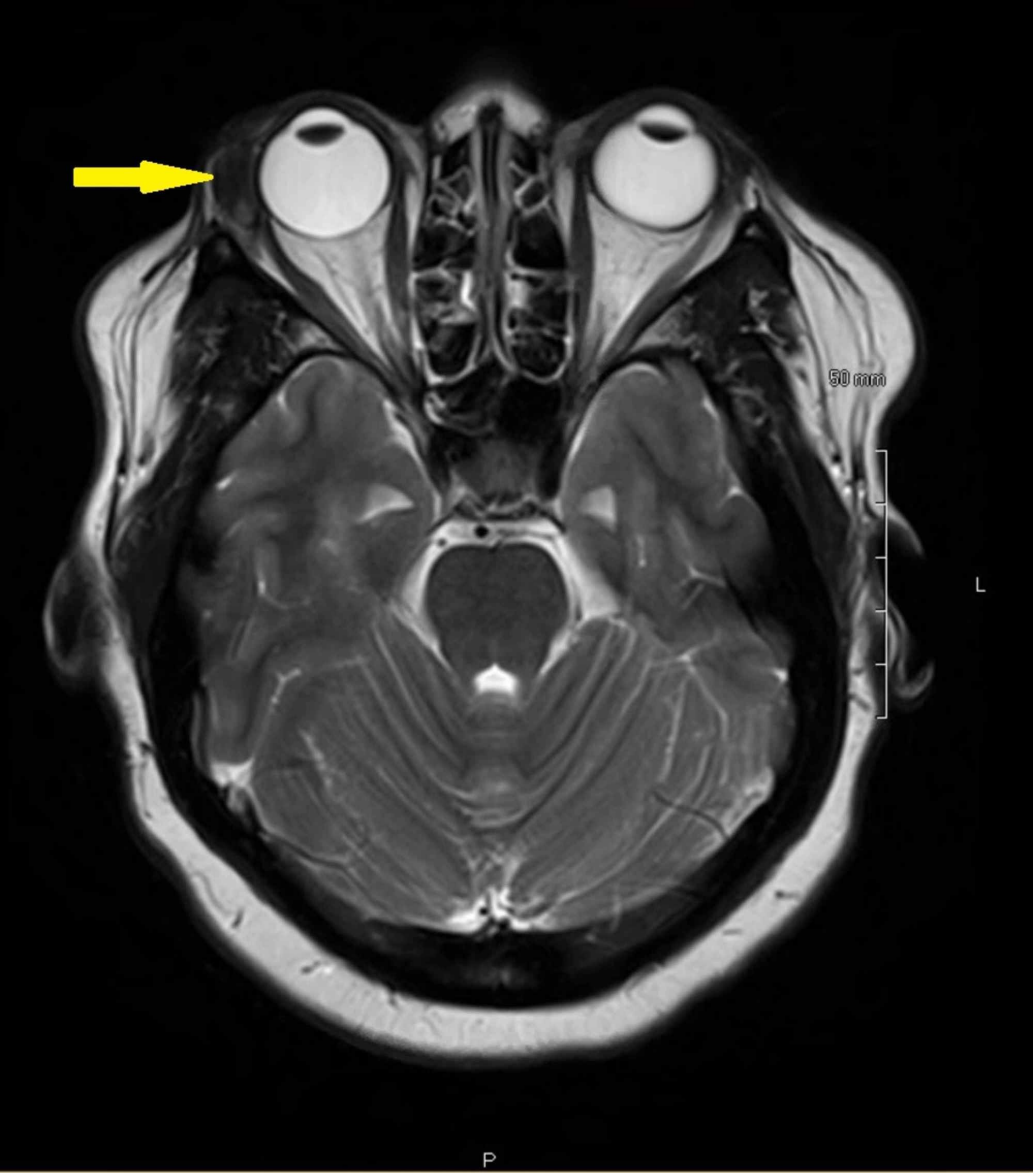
MRI brain and orbit without contrast MRI brain and orbit without contrast. T2 axial sequence showing a 3.1 x 1.6 cm hypointense lesion involving the right lacrimal gland.
